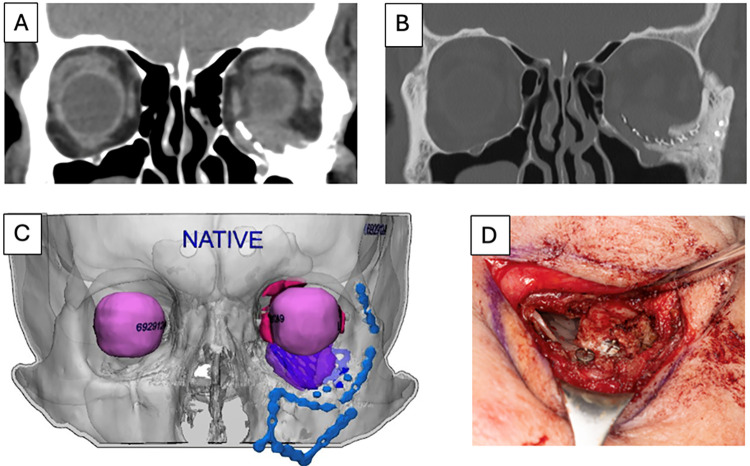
Virtual surgical planning for removal of hardware and capsule. (A) Soft-tissue window coronal CT demonstrates presence of a large capsule and hardware malposition with protrusion into orbital soft tissue. (B) Bone window coronal CT shows heterotopic bone formation surrounding the implant. (C) Digital model displays mass effect of capsule on the globe and precise location of fixation screws. (D) Intraoperative view of capsule, aberrant bone and hardware; the implant and abnormal capsular tissue were removed and no additional hardware was placed, because support for the globe was deemed adequate.
